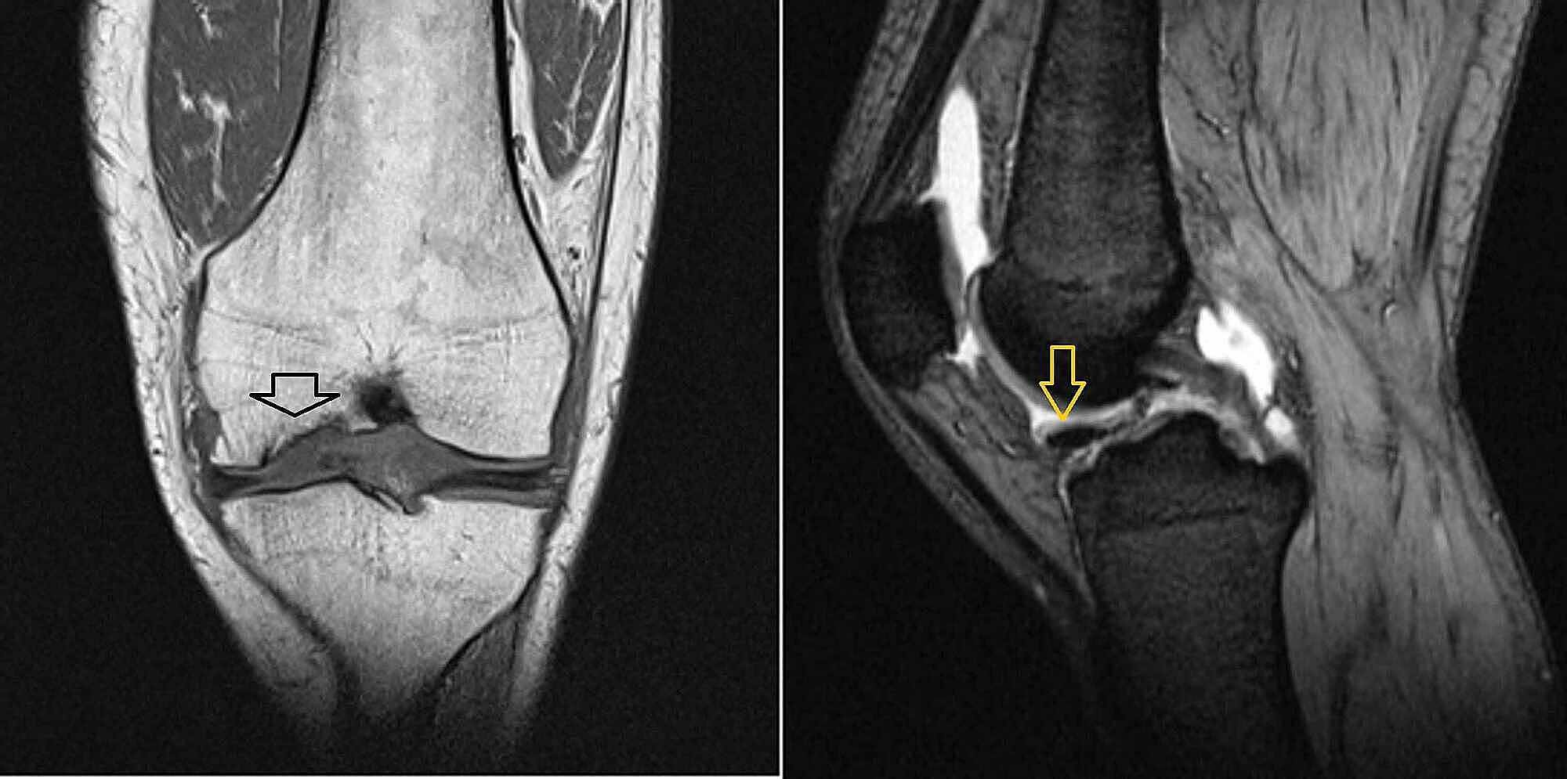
MRI scan (T1 and T2 weighted) revealing osteochondral defect on the weight-bearing surface of the medial femoral condyle and loose body in the anterior knee compartment
